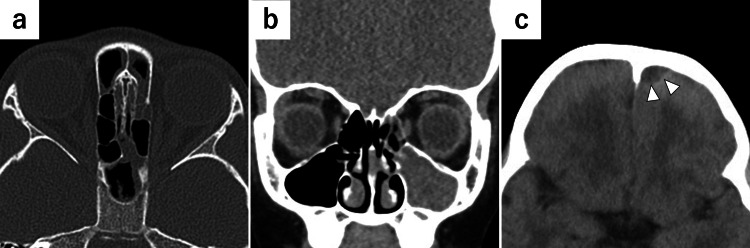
Non-contrast CT images Non-contrast CT images showing (a: axial, b: coronal) soft tissue opacification of the left paranasal sinuses and (c: axial) a low-density area in the left frontal region. No bone defects were observed. CT: computed tomography
